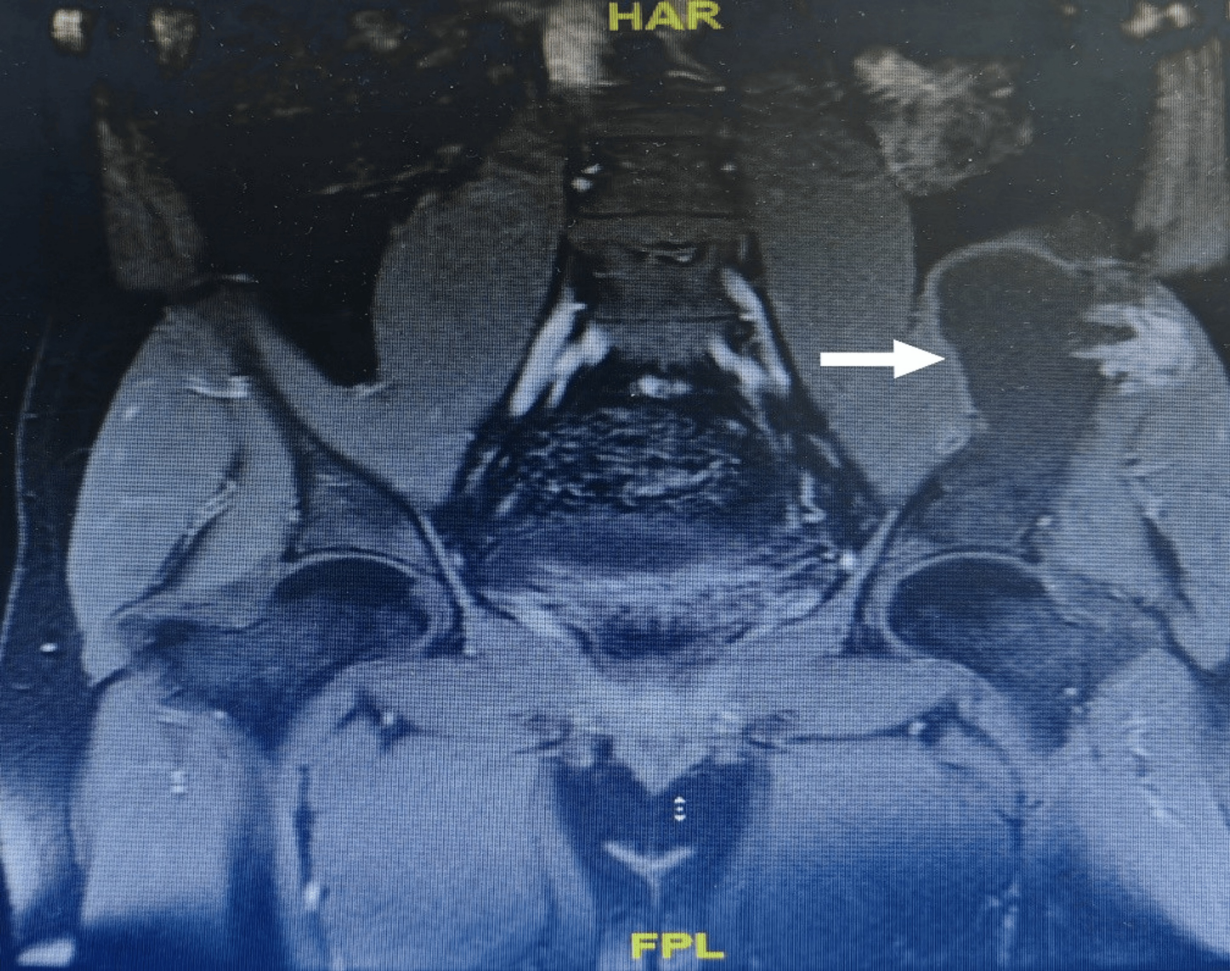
MRI pelvis with intravenous contrast demonstrate left iliac bone lytic lesion with evident of cortical breaching MRI, magnetic resonance imaging
